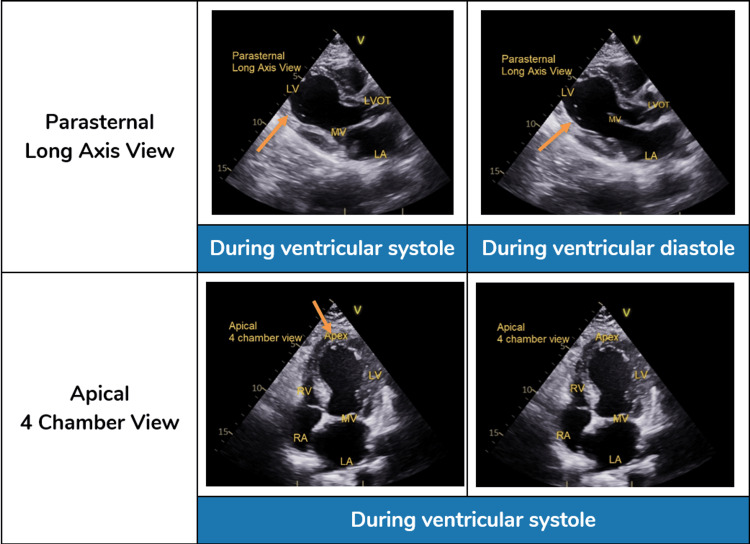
Admission transthoracic echocardiogram demonstrating classic features of takotsubo cardiomyopathy. Initial transthoracic echocardiogram (TTE) performed at admission demonstrated mid-to-distal left ventricular (LV) hypokinesis with apical ballooning, preserved basal contractility, and severely reduced global left ventricular (LV) systolic function (left ventricle ejection fraction: 25–30%), alongside normal left ventricular (LV) dimensions (end-diastolic diameter: 48 mm), mild diastolic dysfunction (E/e’ ratio: 12), and trace mitral regurgitation, hallmarks of takotsubo cardiomyopathy. LV: left ventricle; LA: left atrium; LVOT: left ventricular outflow tract; MV: mitral valve; RA: right atrium; RV: right ventricle.
